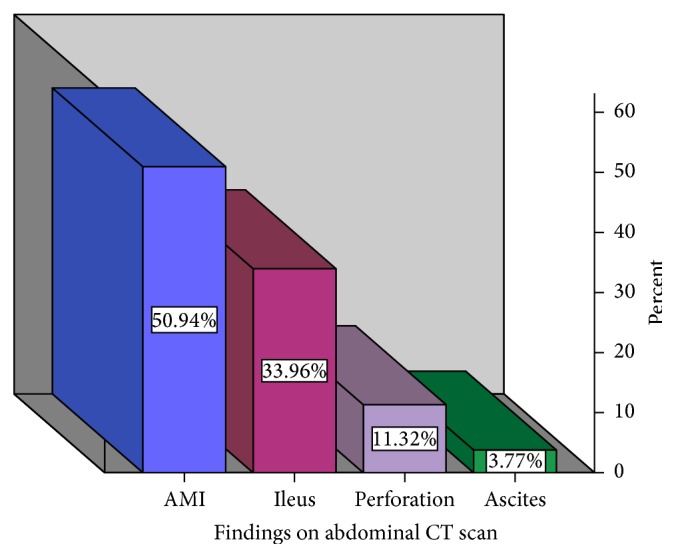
Preoperative CT findings. AMI was confirmed in just about 50% of cases following CT angiography. CT: computed tomography. Multiple findings were present in 40% of cases.
